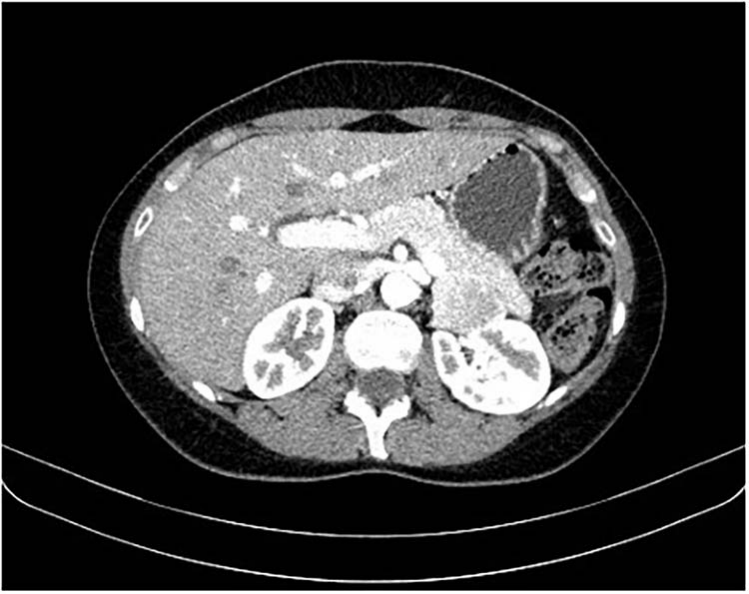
CT scan showing, in addition to signs of chronic liver disease, the presence of a solid subcapsular nodule of segment VII, measuring 23 mm, with hypervascular behavior in the arterial phase.
